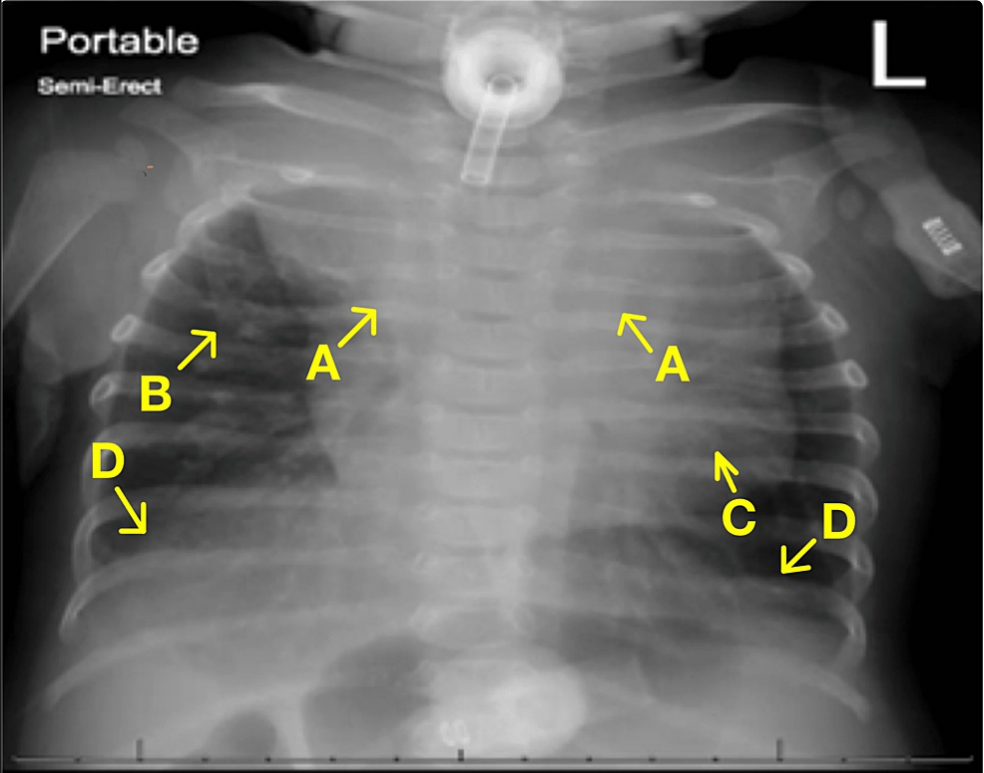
Chest X-ray of the infant on admission to the tertiary pediatric center. (A) Perihilar atelectasis. (B) Interstitial edema. (C) Cardiomegaly with enlarged cardio-thymic silhouette. (D) Bibasilar atelectasis.
